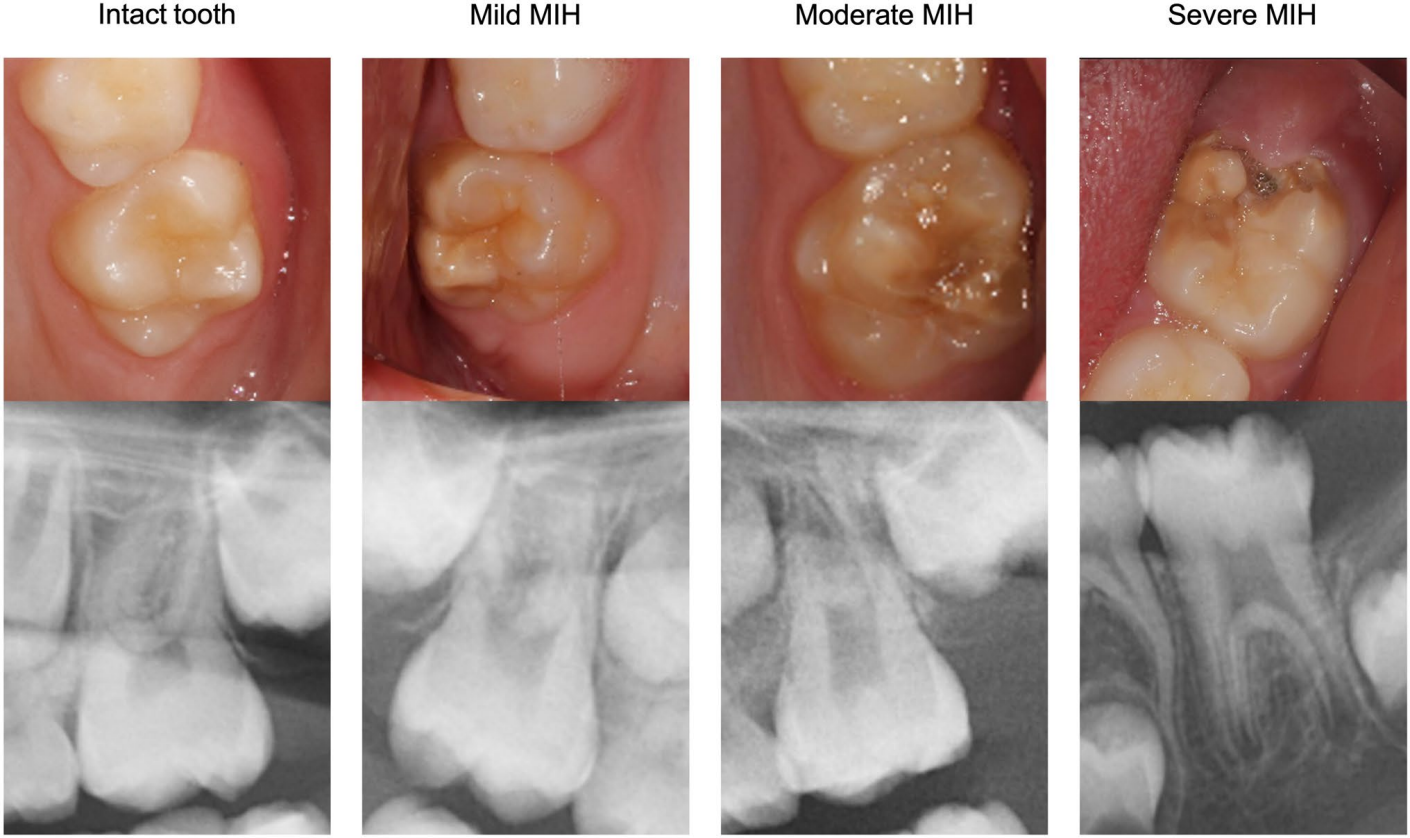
Simplified index of grading each first permanent molar, clinical and radiographic assessment. Intact tooth with sound enamel, mild MIH with demarcated opacities without enamel breakdown, moderate MIH with demarcated opacities with enamel breakdown or atypical restauration of ≤ 2 surfaces, and severe MIH with demarcated opacities with enamel breakdown or atypical restauration, involving > 2 surfaces and/or extensive lesion
